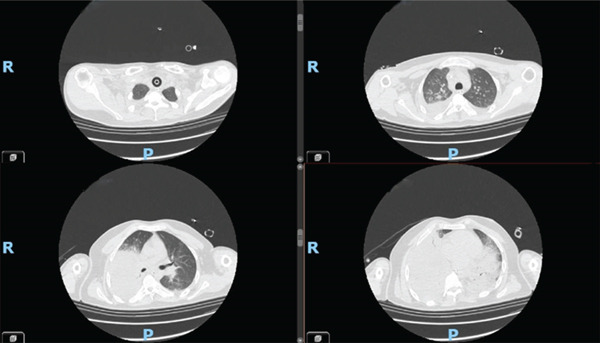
CT scan performed on admission.
